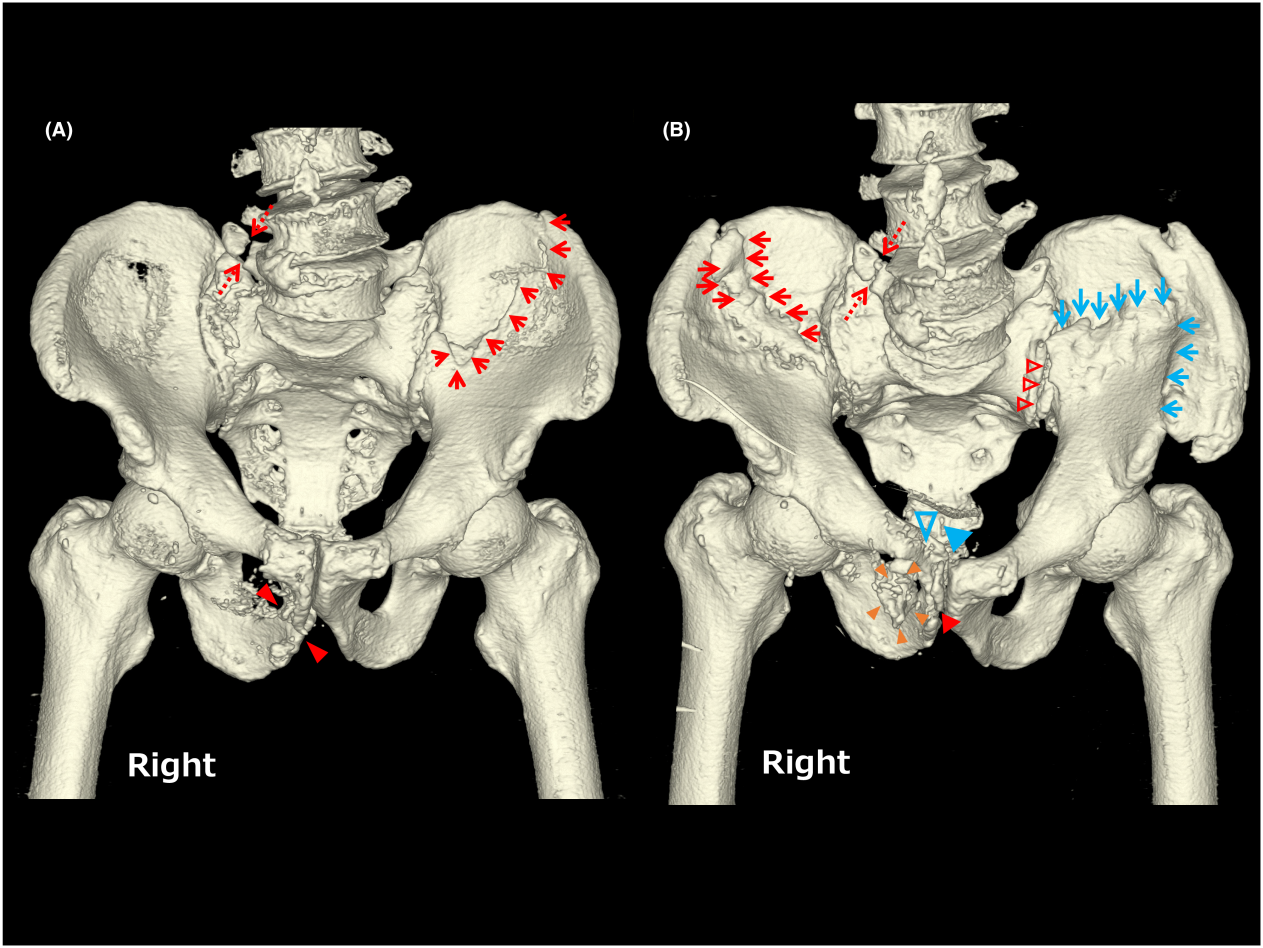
A and B show the frontal 3D computed tomography reconstruction of the abdominopelvic computed tomography (CT). A was taken 6 months before admission and incidentally revealed pelvic fractures of the left ilium (red arrows), right costal process of the fifth lumbar vertebra (red dotted arrows), and right ischium (red arrowheads), but no dislocation of the pelvic ring. In contrast, B was taken on admission when CT‐guided catheter‐inserted drainage of the intermuscular abscesses was performed. It revealed fragility pelvic fractures with new fracture sites and pelvic ring disruption: right ilium (red arrows), deviated left ilium (blue arrows), right costal process of the fifth lumbar vertebra (red dotted arrows), left sacrum (red open arrowheads), deviated right ischium (red arrowhead), detached right ischium (dark yellow arrowheads), right pubis (blue open arrowhead), and pubic symphysis diastasis (blue arrowhead).
